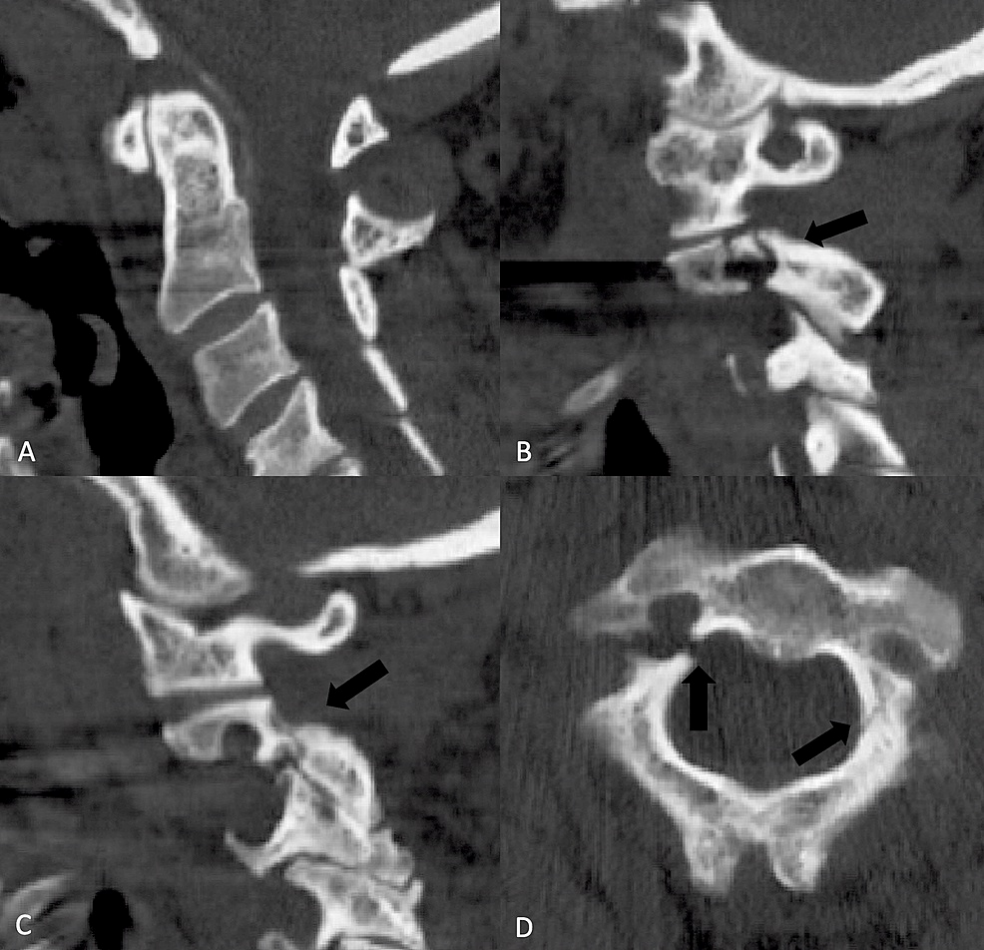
A CT scan of the cervical spine of a healthy, 61-year-old woman following trauma demonstrating fracture though the par interarticularis on the right (B) and left (C). The midsagittal cut (A) showed no evidence of malalignment. Note the very small dimensions of the C2 pedicles seen on the axial image (D) that were judged to be anatomically unsuitable for direct repair surgery. CT: computed tomography
